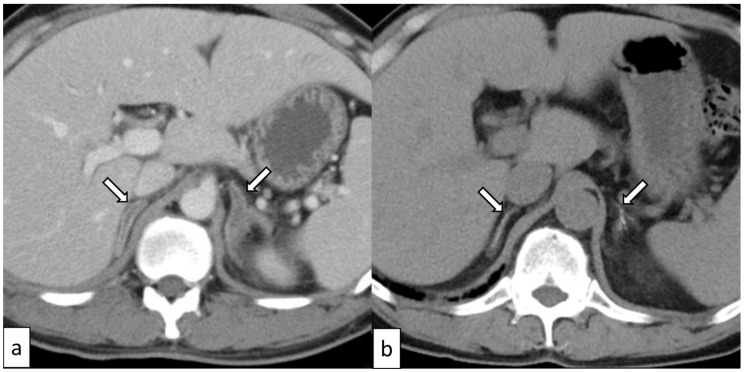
A 38-year-old man with TAFRO syndrome. A contrast-enhanced CT scan shows bilateral adrenomegaly and poorly enhanced adrenal glands ((a), arrows). One month later, the atrophy and calcification of the affected adrenal glands appear on a non-enhanced CT scan ((b), arrows).
